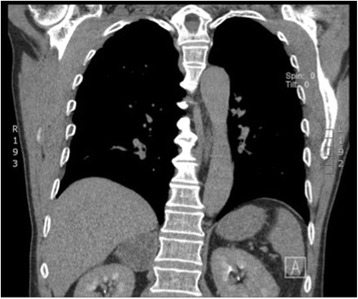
Computed tomography image demonstrates incidentaloma of 6 cm in the right adrenal gland
